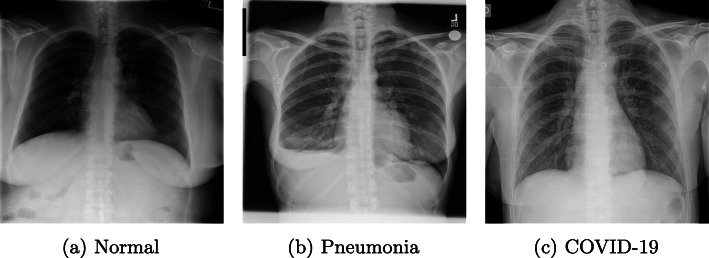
Representative examples of chest X-ray images of normal (patient without pulmonary conditions), patient with pneumonia (others than COVID-19) and patient with COVID-19
